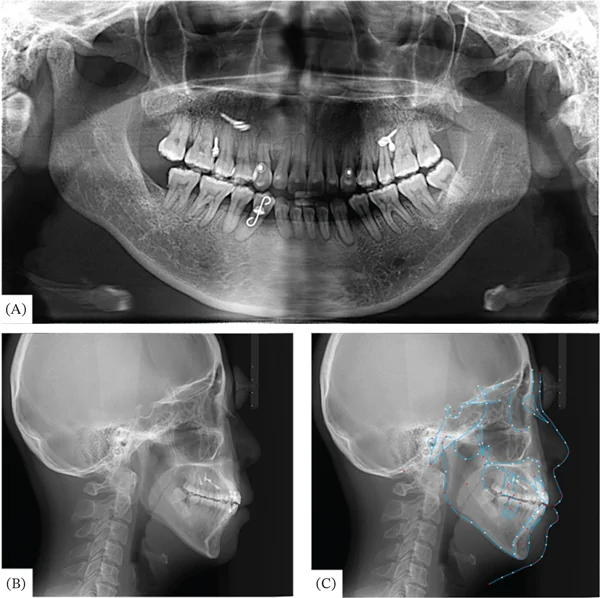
On‐treatment radiographs. (A) Panoramic radiograph, (B) lateral cephalogram, and (C) lateral cephalogram tracing.
